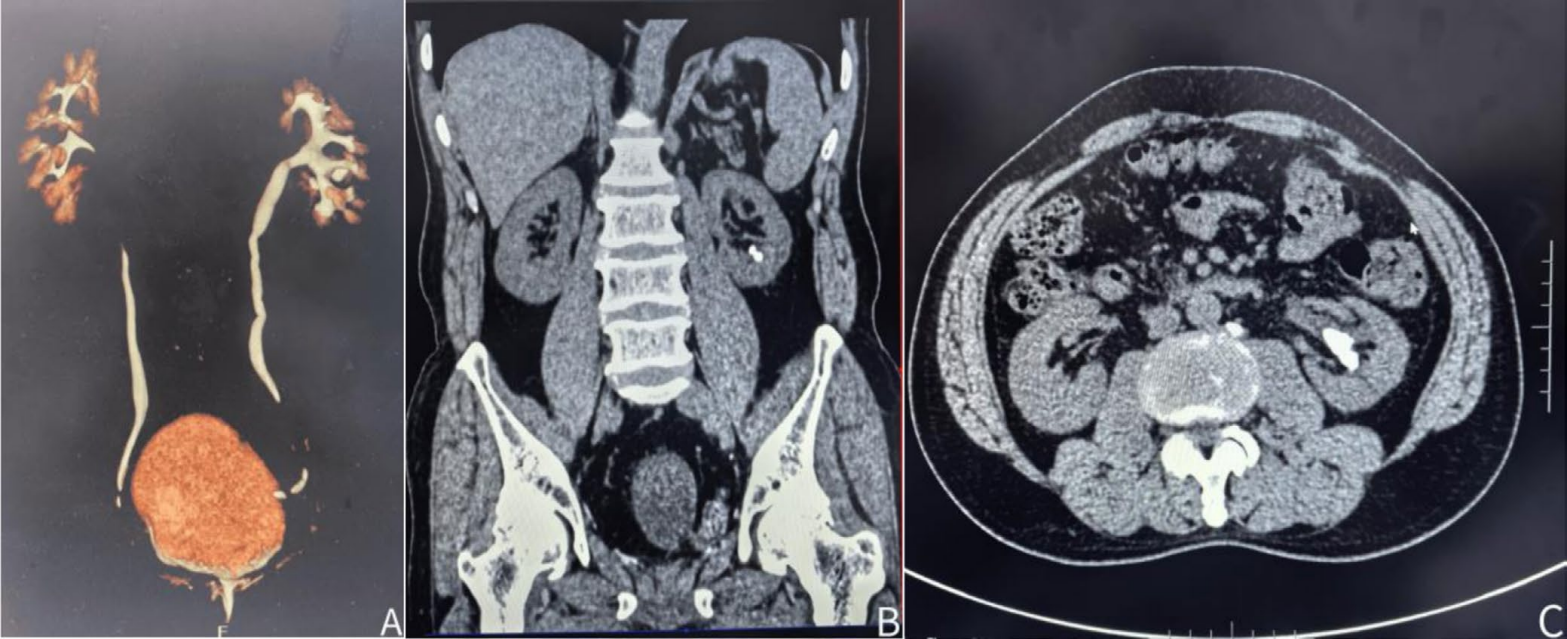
Preoperative computed tomography urography, (A) Three‐dimensional reconstruction demonstrates the ureteral orifice positioned at the bladder apex, (B) Sagittal view reveals the renal calculus in the lower calyceal, (C) Coronal view of CTU shows the calculus located in the renal pelvis.
